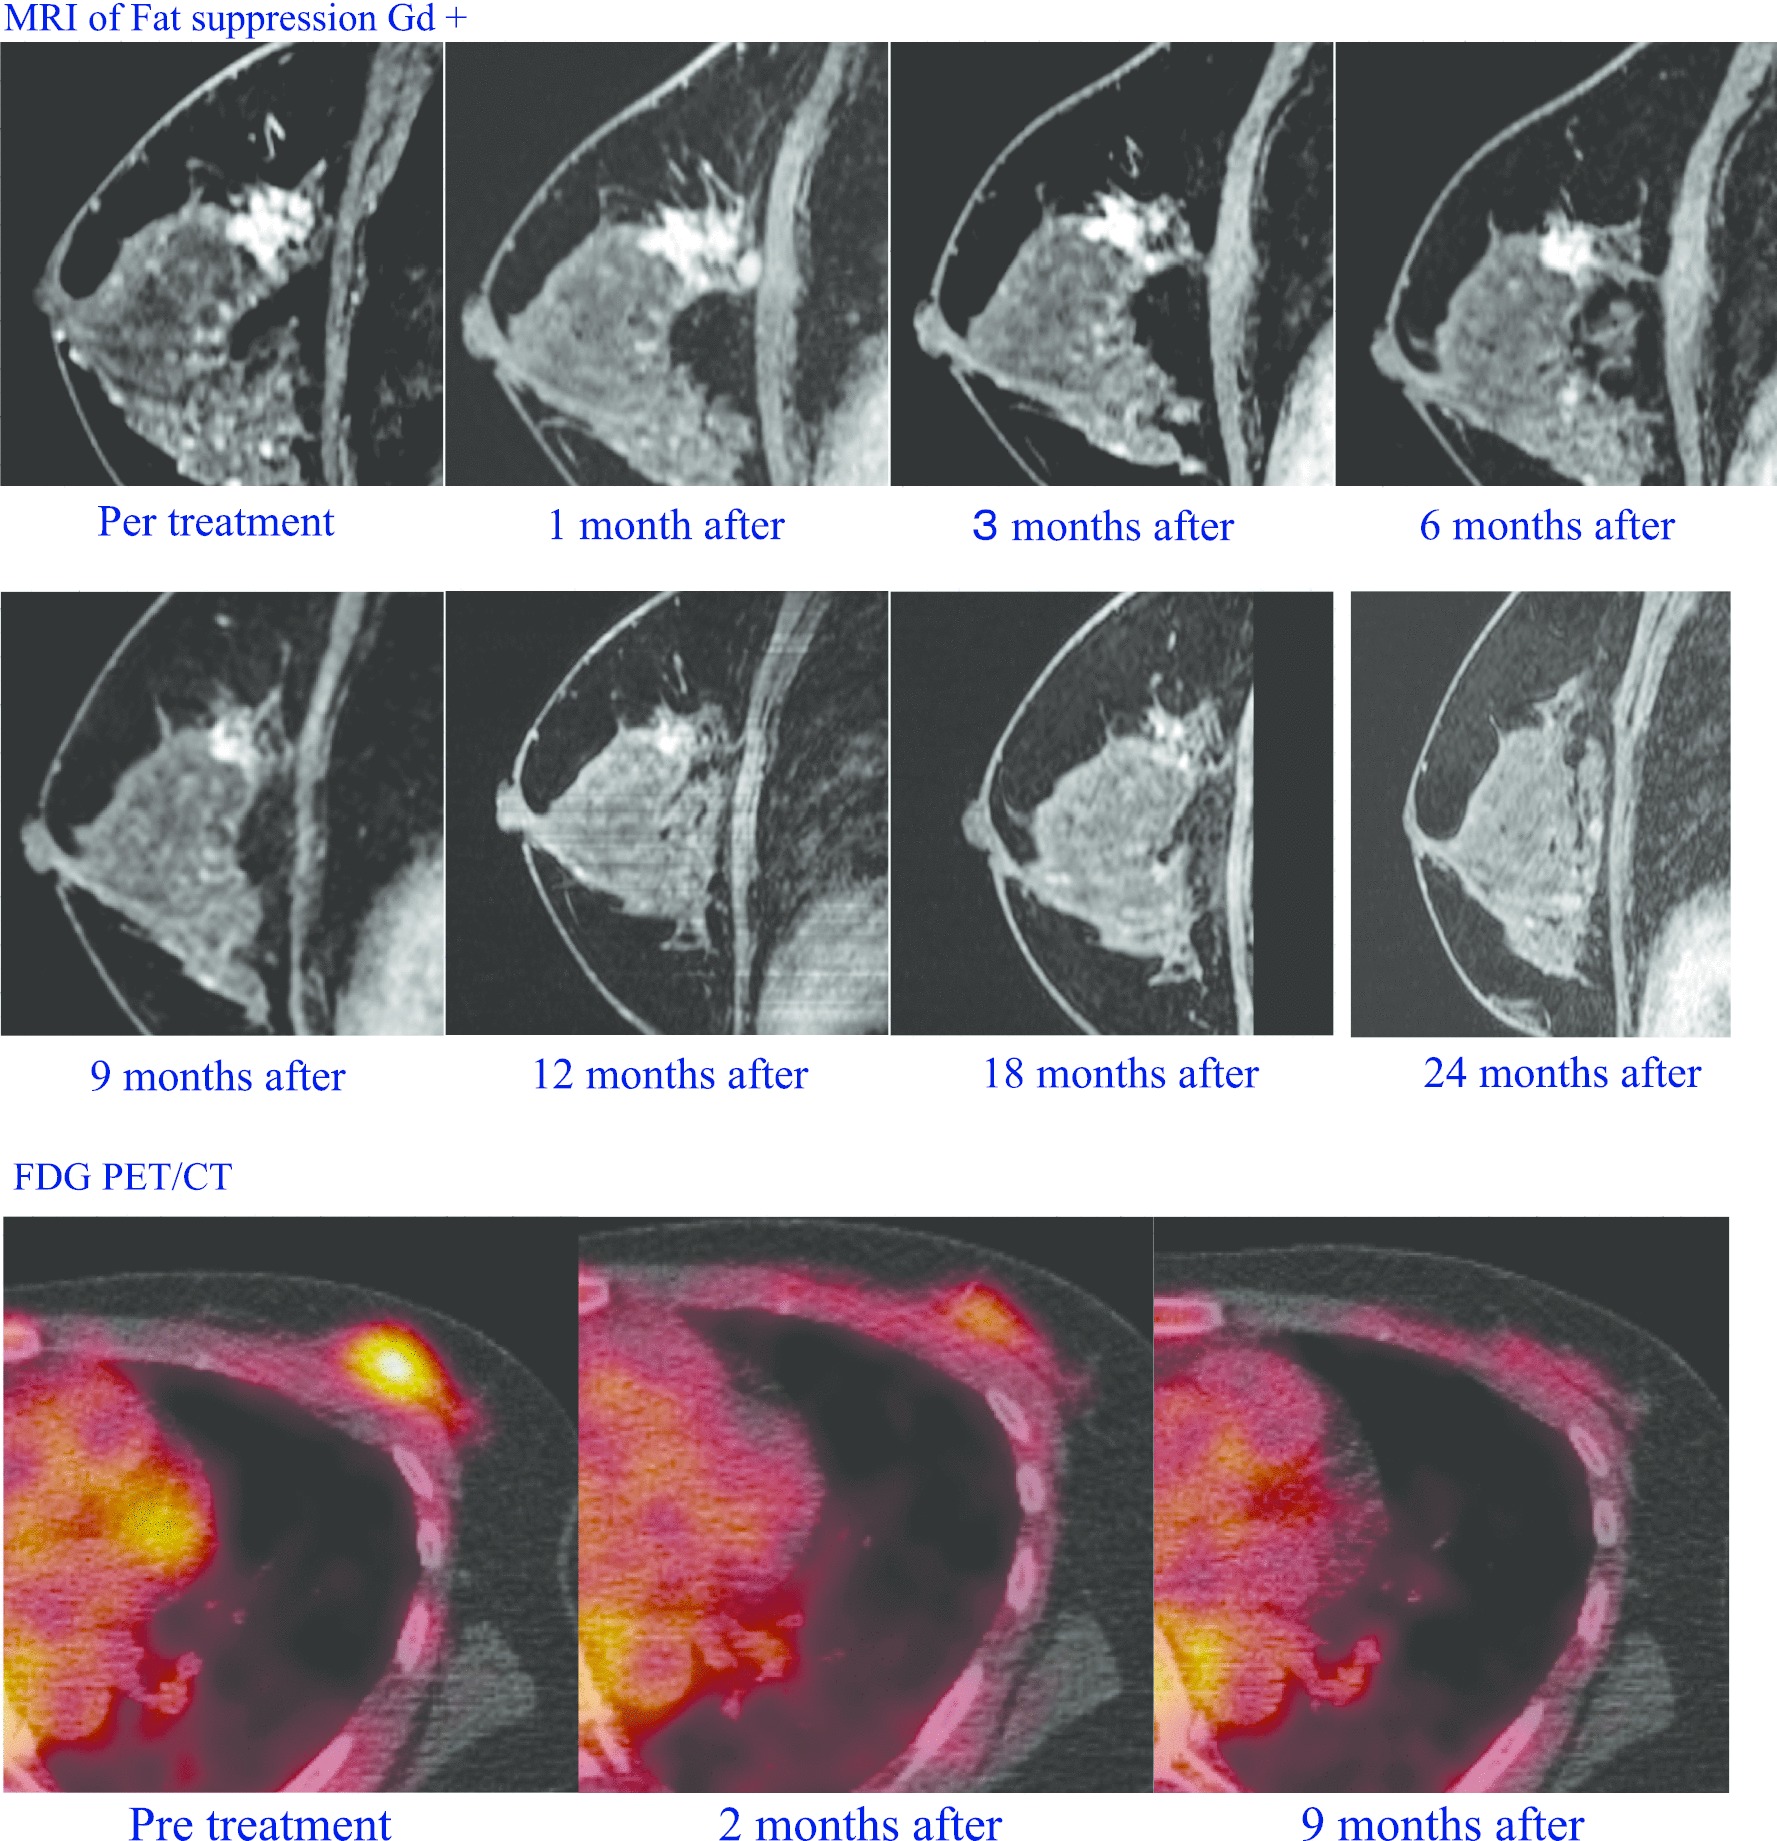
MRI and FDG-PET image of the first case. A 50-year-old female with 20-mm left breast cancer (ER+, PgR-, HER2–) was treated with 52.8 Gy (RBE) C-ion RT and adjuvant 5-year tamoxifen (TAM) and 2-year LH-RH agonist. Contrast-enhanced gradient-echo T1-weighted MR images of pre-treatment, 1 month after, 3 months after, 6 months after, 12 months after and 24 months after C-ion RT. FDG-PET/CT images show the left breast tumor with maximum standardized uptake value (SUV) of 2.5 before treatment. Two months after C-ion RT, FDG uptake was almost normal. At 9 months FDG uptake had disappeared
